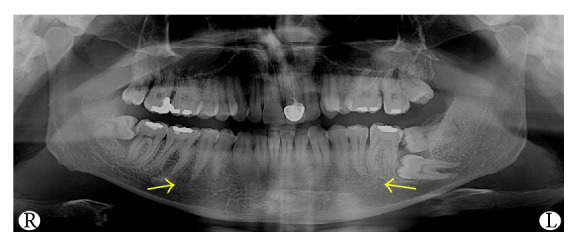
20-year-old patient's panoramic radiograph in which the images of the right MF and the end of the left mandibular canal are pointed by the arrows.
